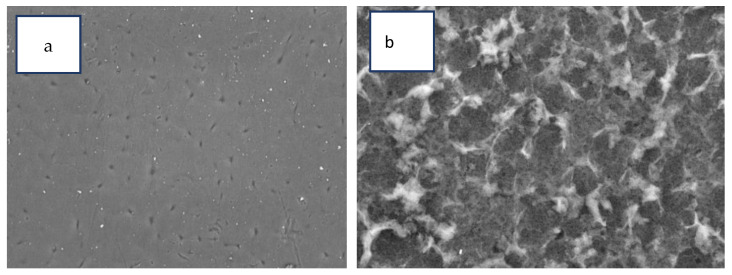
SEM images (magnification 4000) of (a) CTA/0.3%GO substrates and (b) the PA-TFC membrane.
